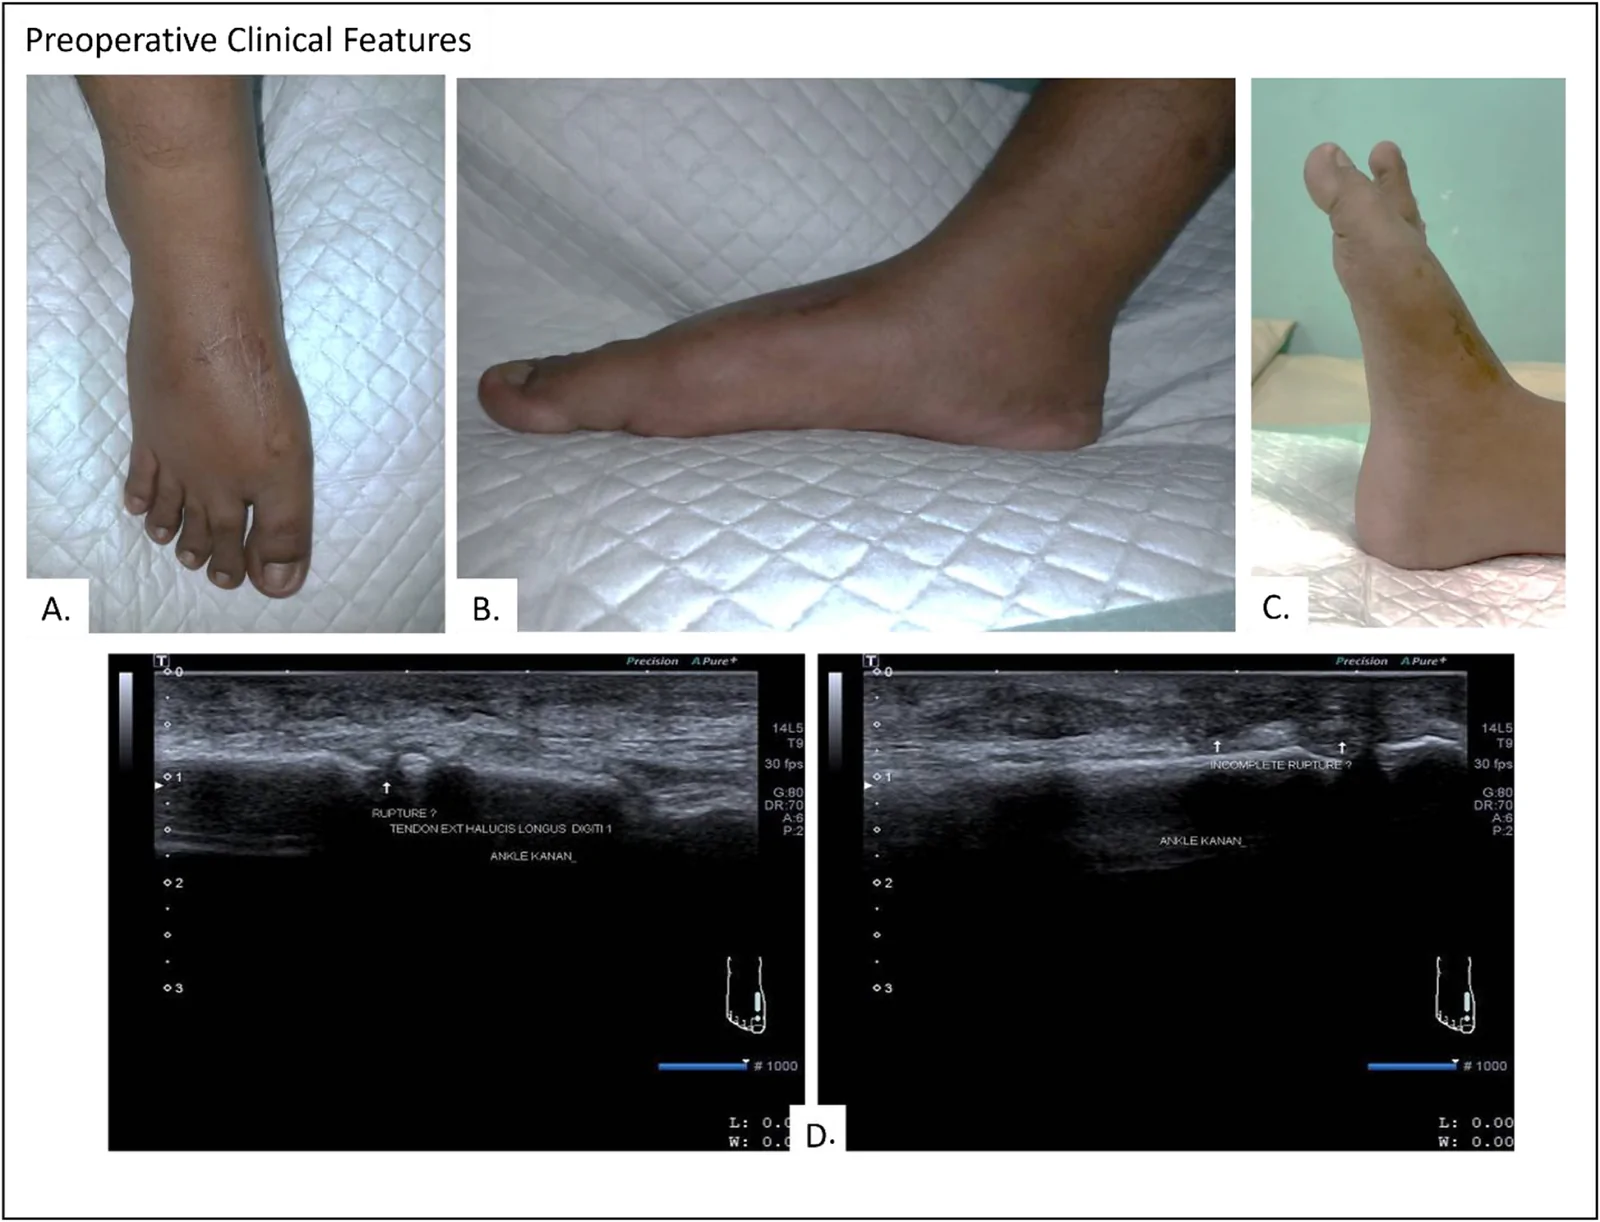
Preoperative clinical images: (A) Dorsal aspect of the foot; (B) Lateral aspect of the foot; (C) Lateral aspect of the foot with a dropped great toe; (D) Ultrasound evaluation of the EHL rupture.
